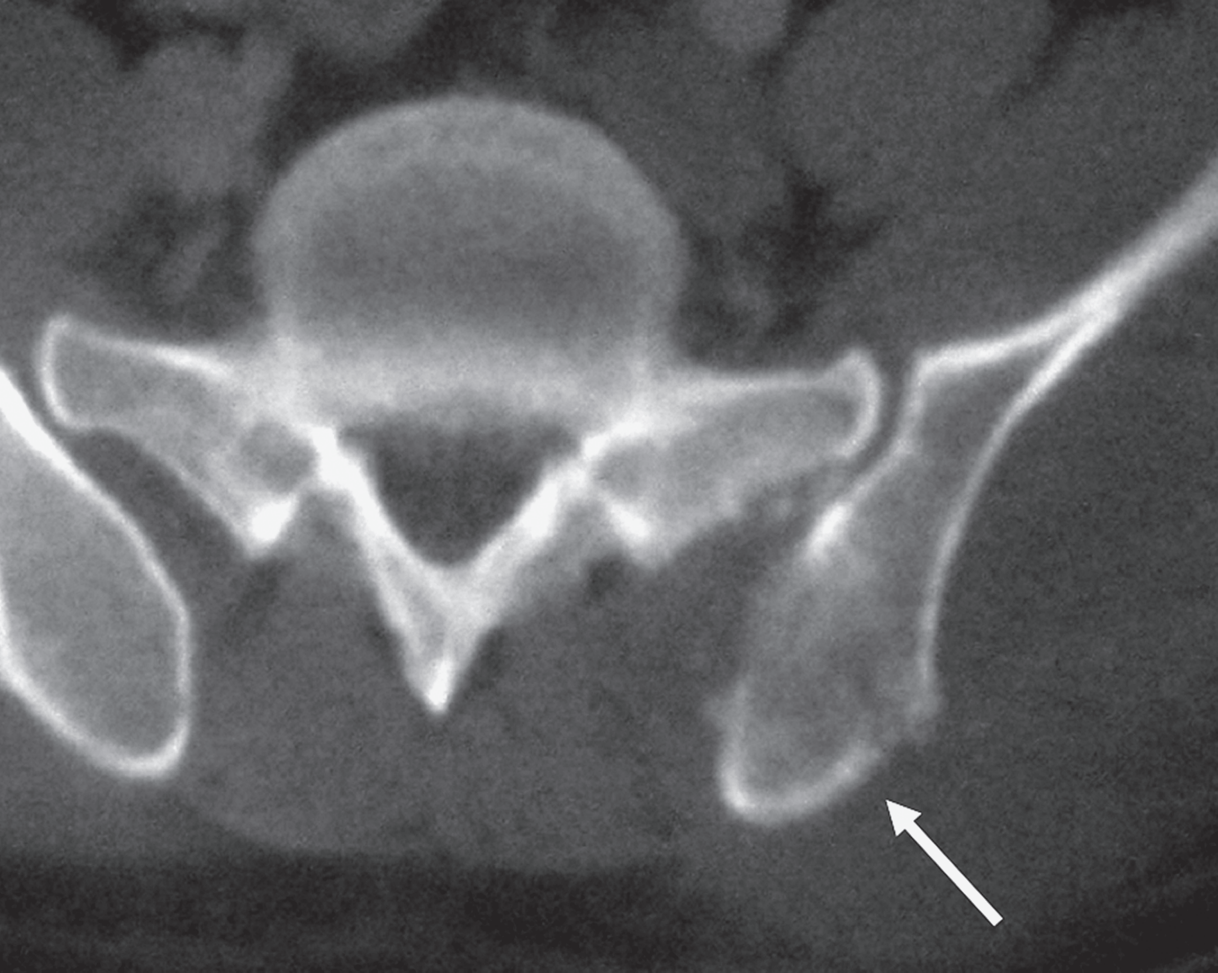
Case 2. Plain CT showed a lytic lesion in the posterior superior iliac spine (arrow).
